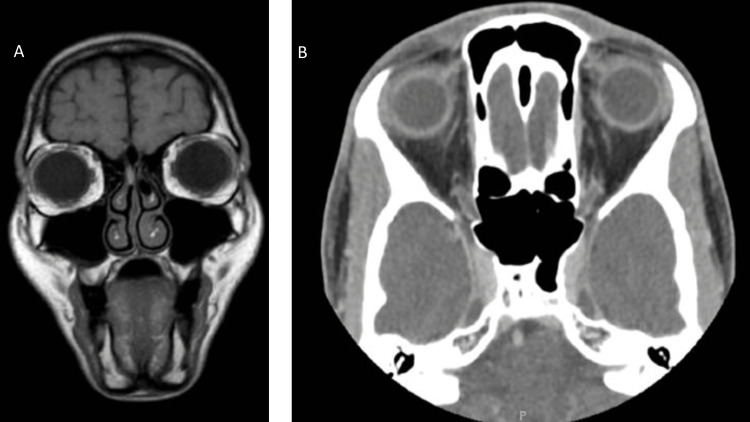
Radiographic image of the lesion MRI T1 coronal view (A) and CT scan axial view (B) showed a hyper-intense lesion in the right olfactory recess and right nasal cavity roof associated with thinning of the right cribriform plate with no involvement of the paranasal sinuses.
